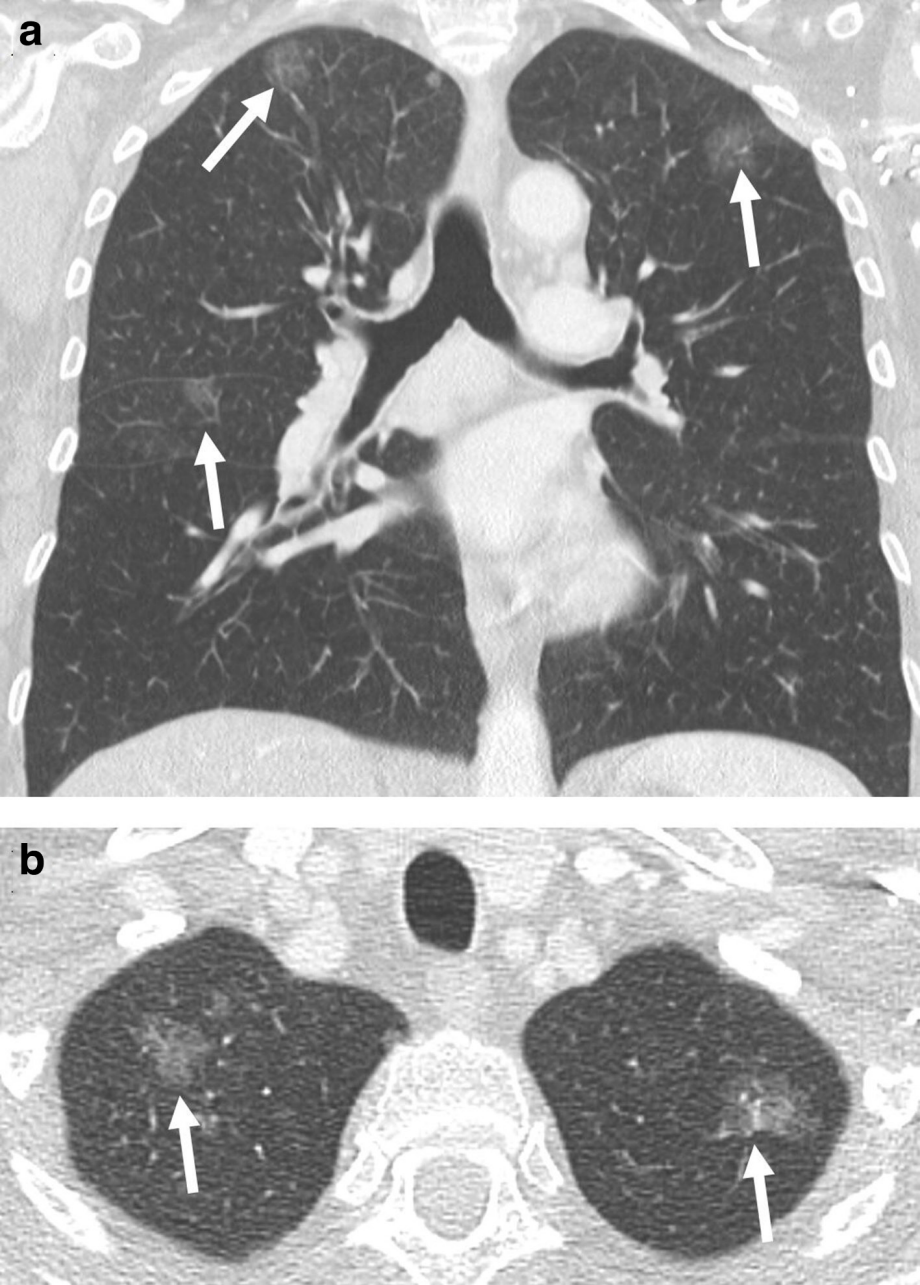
(a) Coronal and (b) axial contrast-enhanced CT of a female patient with three pure ground-glass nodules that represent *in situ* adenocarcinomas in the right and left upper lobe, as well as the middle lobe (arrow). The correct staging is based on the tumour dimension of the largest ground-glass nodules (3.3 cm), as well as the number of tumours, which is given in brackets: T2a(3)N0M0.
